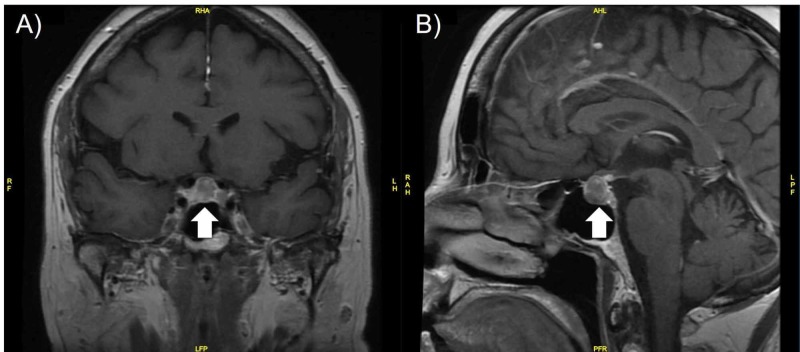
MRI T1-FLAIR images of the pituitary tumor prior to transsphenoidal resection. Images reveal an inhomogeneously enhancing pituitary tumor measuring 10 mm size with suprasellar extension and contacting the inferior aspect of the optic chiasm (arrow). (A) Coronal view; (B) Sagittal view.
